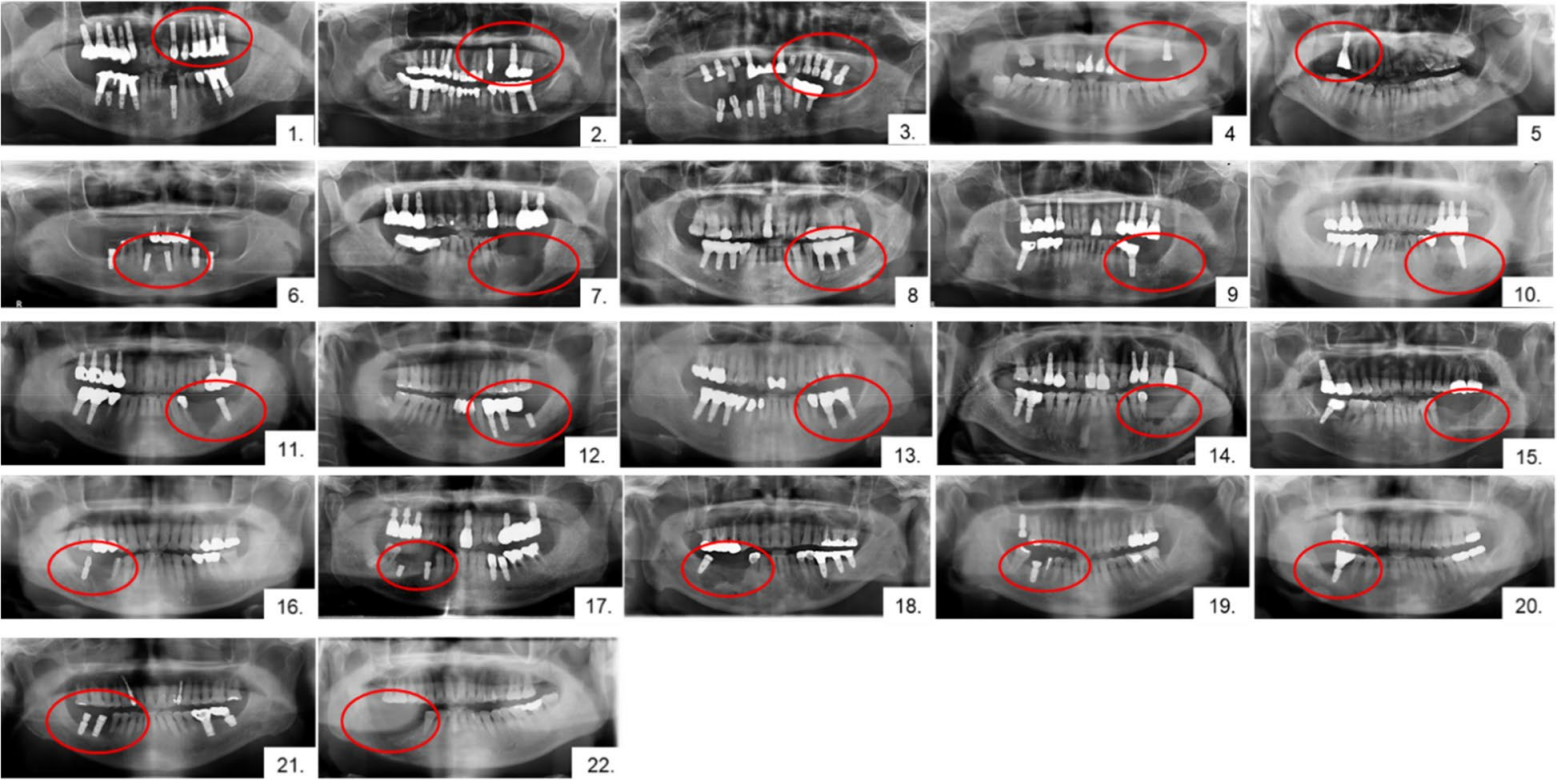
Panoramic radiograph at first visit. The red circle indicates the site of OSCC occurrence. Most cases showed marginal alveolar bone loss around the dental implant, whereas some cases (e.g., cases 1, 6, 12, 19) did not show definite bone loss
